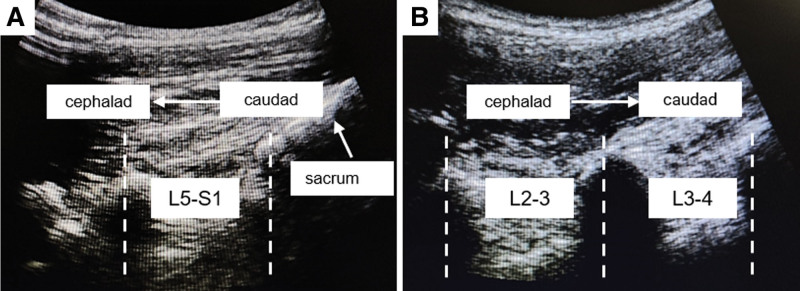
Ultrasound-assisted positioning. (A) The sacrum and L5-S1 interspace were positioned using sliding and tilting scanning techniques in paramedian sagittal oblique orientation with a curved array transducer and the L4-5, L3-4, and L2-3 interspace was successively located by moving the transducer cephalad. Arrowhead indicated sacrum. (B) The T12 vertebra was identified at its joint with the 12th rib and the transducer was moved caudad to identify each consecutive interspace.
